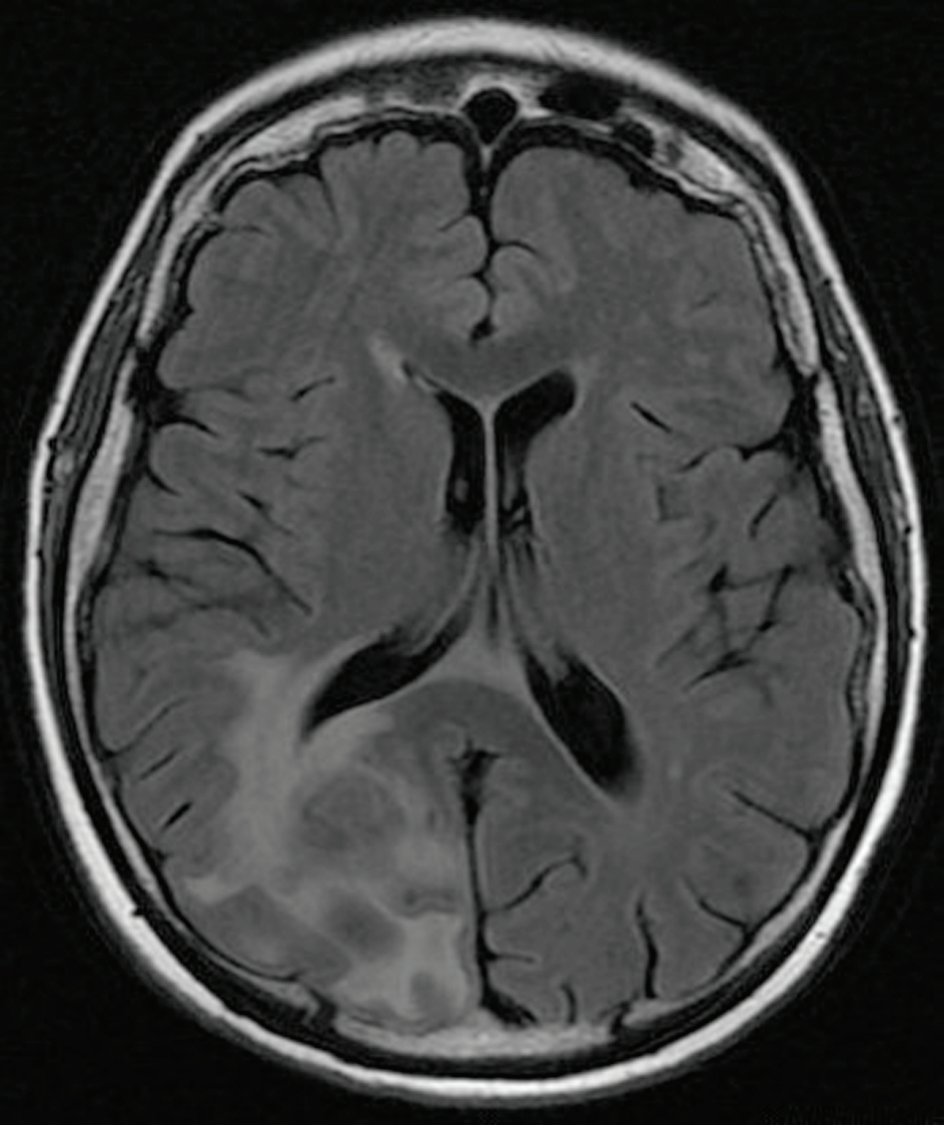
Pre-operative brain MRI FLAIR sequence which shows the peritumoral edema.
